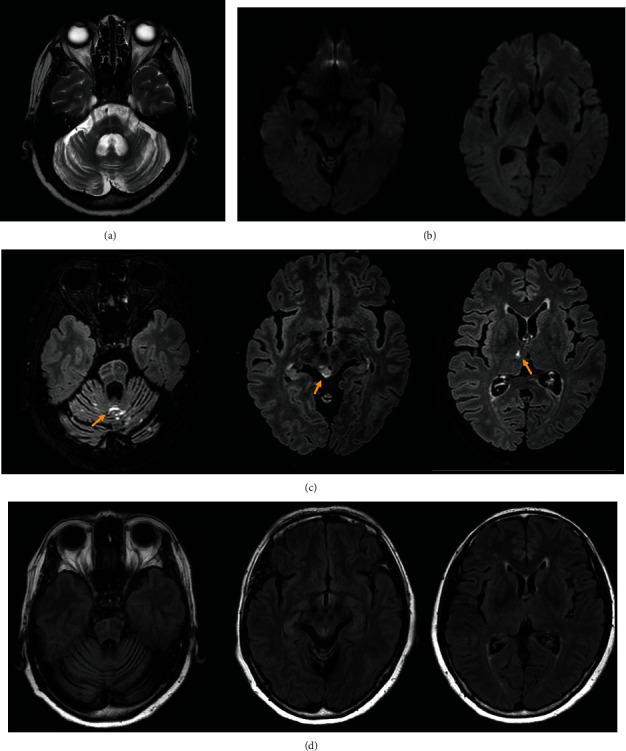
Brain magnetic resonance imaging (MRI) findings in patient S-103. (a) T2-weighted images. The brain MRI revealed findings consistent with multiple system atrophy including atrophy in the pons, middle cerebellar peduncle, and cerebellum. (b) Diffusion-weighted images on the day following the intra-arterial mesenchymal stem cells. Acute ischemic lesions were not observed. (c) Fluid-attenuated inversion recovery (FLAIR) with enhancement images on the day following the intra-arterial mesenchymal stem cells. Leptomeningeal enhancement occurred in the cerebellar folia along with enhancement in the right superior colliculus and right thalamus (yellow arrows). (d) FLAIR images four days after the procedure. The follow-up brain MRI demonstrated resolved hyperintensity in the right superior colliculus and right thalamus.
